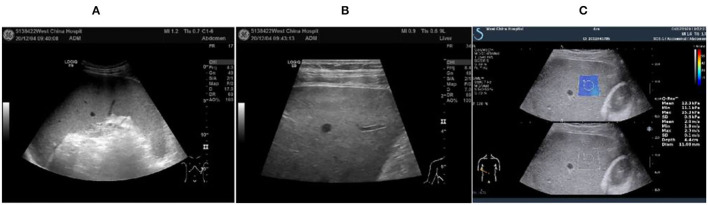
Abdominal ultrasound image of the 47-year-old at 2020.12.04. (A) Intercostal spleen thickness was ~7.0 cm and parenchymal echo was uniform; (B) Hepatomegaly with liver parenchymal damage; (C) Liver hardness measurement: 12.3 kpa.
